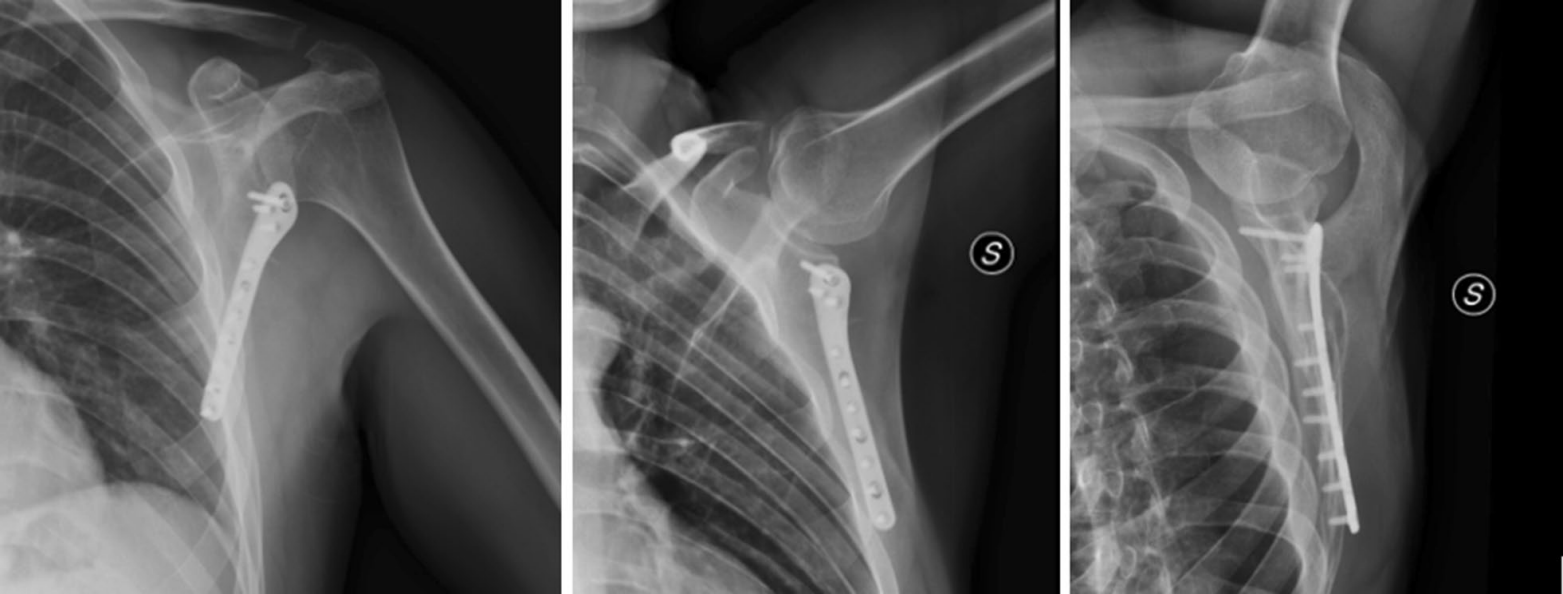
Lateral border plate (X-ray 2 years after operation)
